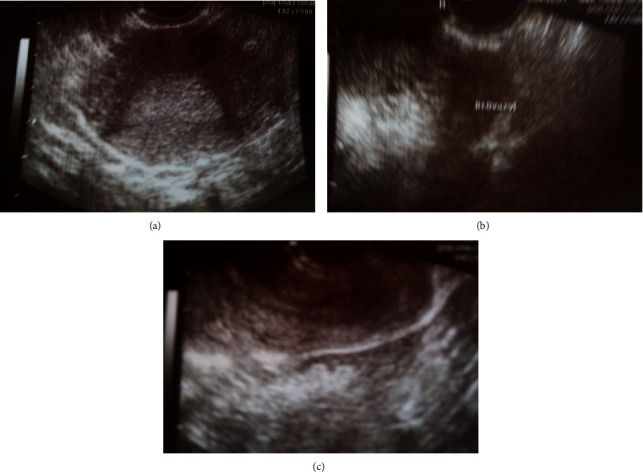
(a) Transvaginal ultrasound: the absence of a gestational sac in the endometrial cavity supports the diagnosis of an ectopic pregnancy (our case). (b) Transvaginal ultrasound: the presence of mass in the anatomical area of the adnexa supports the diagnosis of an ectopic pregnancy (our case). (c) Transvaginal ultrasound: the presence of blood clots in the pouch of Douglas supports the diagnosis of an ectopic pregnancy (our case).
